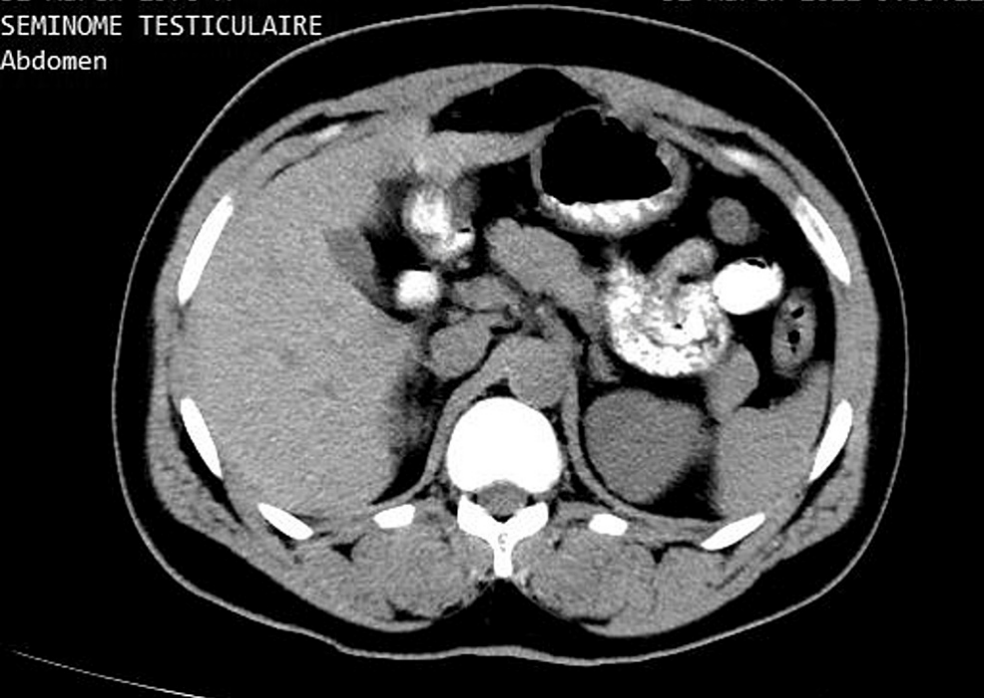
Computed tomography section of the abdomen at the level of the proximal renal arteries showing no metastases to the para-aortic lymph nodes
